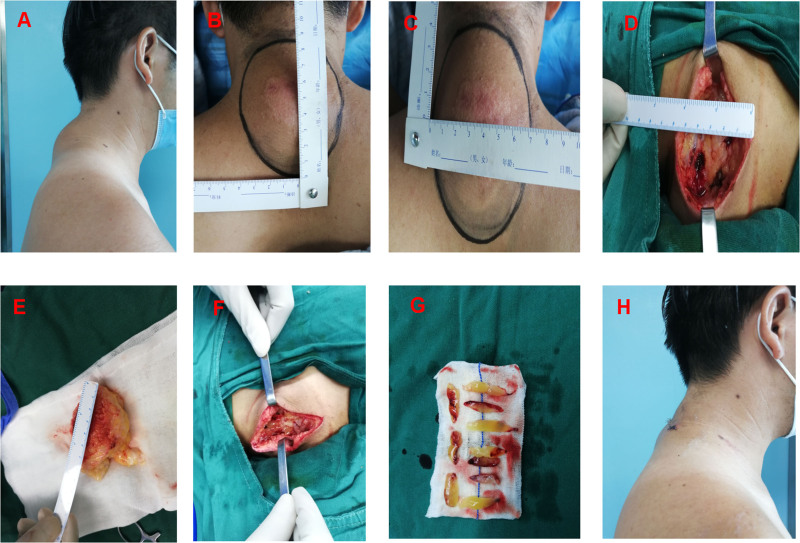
A 45-yr old male patient was treated with concentrated growth factor gel to fill the wound cavity after resection of a huge tumor in the shoulder and neck. (A) Preoperative; (B and C) ultrasound localization, marking of tumor size and resection range; (D) residual wound cavity after tumor resection; (E) excised tumor; (F) intraoperative concentrated growth factor gel was used to fill the wound cavity; (G) concentrated growth factor gel; (H) 7 days after surgery.
